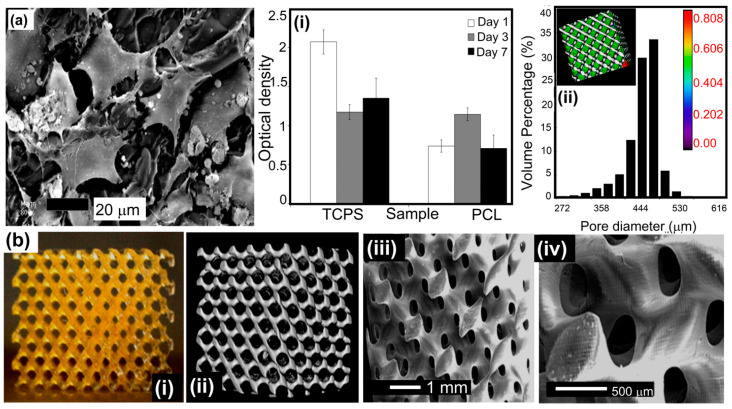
(a) SEM image: fibroblasts after 7 days in a cell culture showing crosslinked PCL, (i) the activity of cells’ metabolism while grown on TCPS (positive control) and photo-crosslinked PCL networks, and (ii) pore size distribution throughout the porous scaffold via μ-CT. (b) Photographs of (i) a micro-computed tomography visualization and (ii) SEM of a scaffold via SLS using a 1500-m macromer (iii,iv) An enlarged view of the open and linked nature of the porous structure indicates that the scaffold exhibited suitable external and internal surfaces. Reprinted with permission [111].
